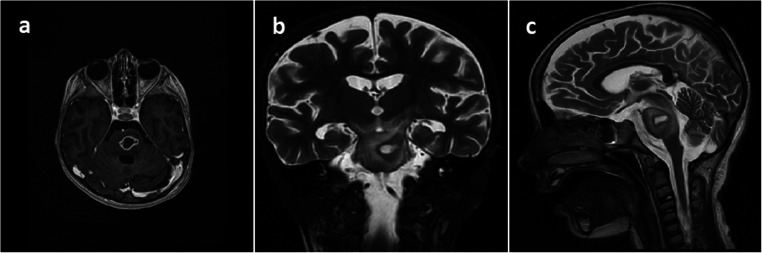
MRI performed 3 days after biopsy a horizontal with gadolinium, b frontal T2-weighted, and c sagittal T2-weighted showing reduction of abscess volume, but the enlargement of surrounding encephalitis area
